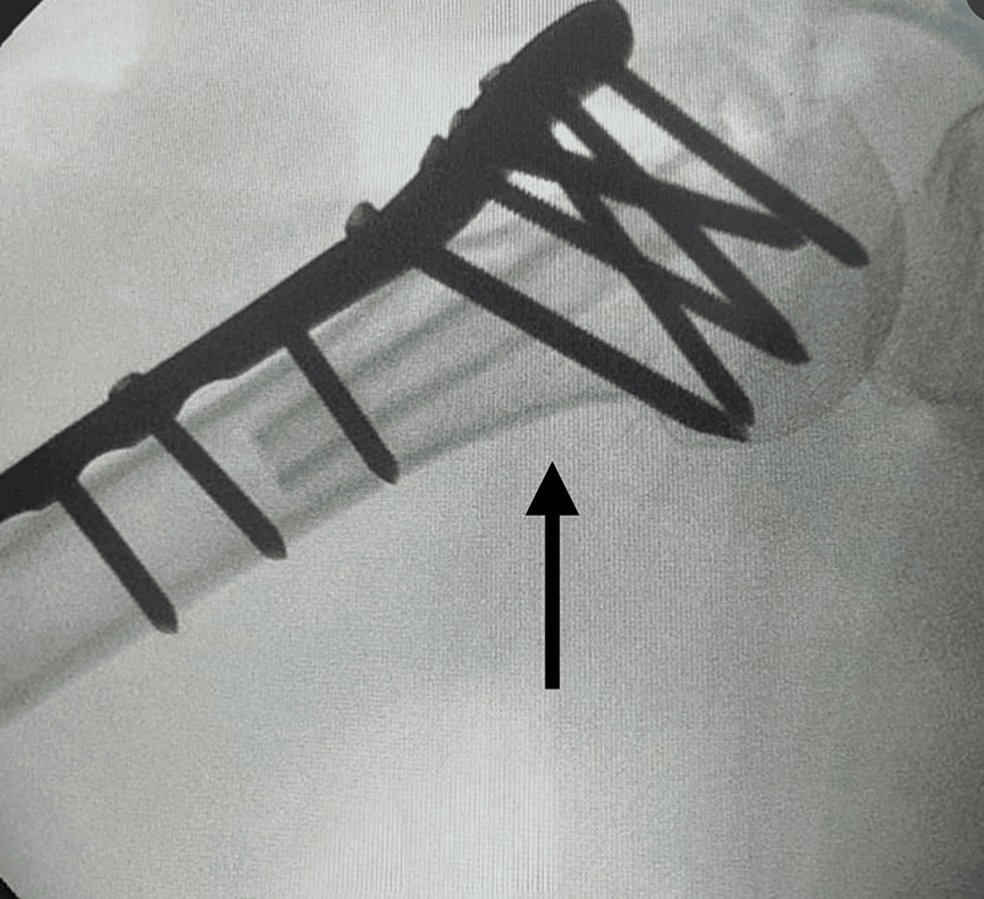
X-ray AP view of the right proximal humerus postoperatively The arrow shows postoperative results: fracture reduction, plate, graft, and screw placement with visible 6.5 cm of fibular autograft in the humerus shaft canal.
